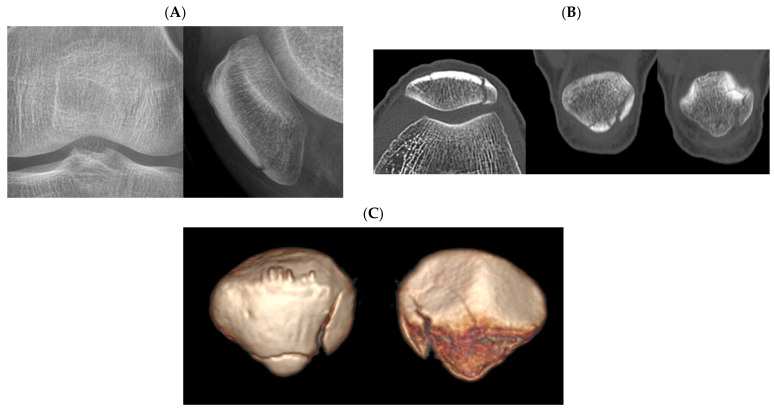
Plain radiographs (A), two-dimensional computed tomography (B), and three-dimensional computed tomography (C) of a patellar fracture with fracture lines of both horizontal and vertical fracture lines. The respondents chose either type A or B.
